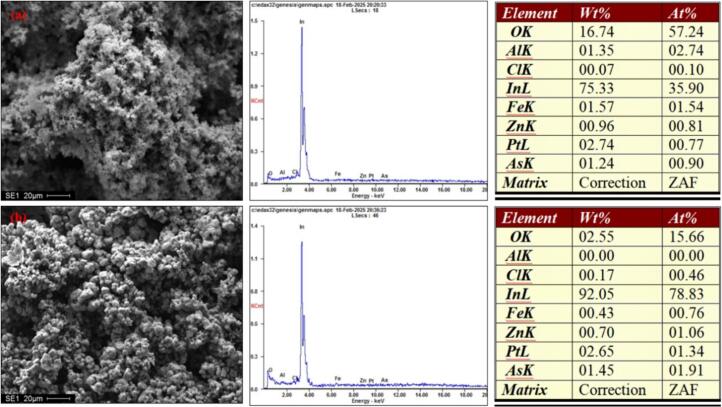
EDS of the deposited layer. a. Conventional electrodeposition; b. Ultrasound-assisted electrodeposition; Deposition potential: −0.80 V, Deposition time: 30 min, Temperature 25℃, ultrasonic power 100 W.
